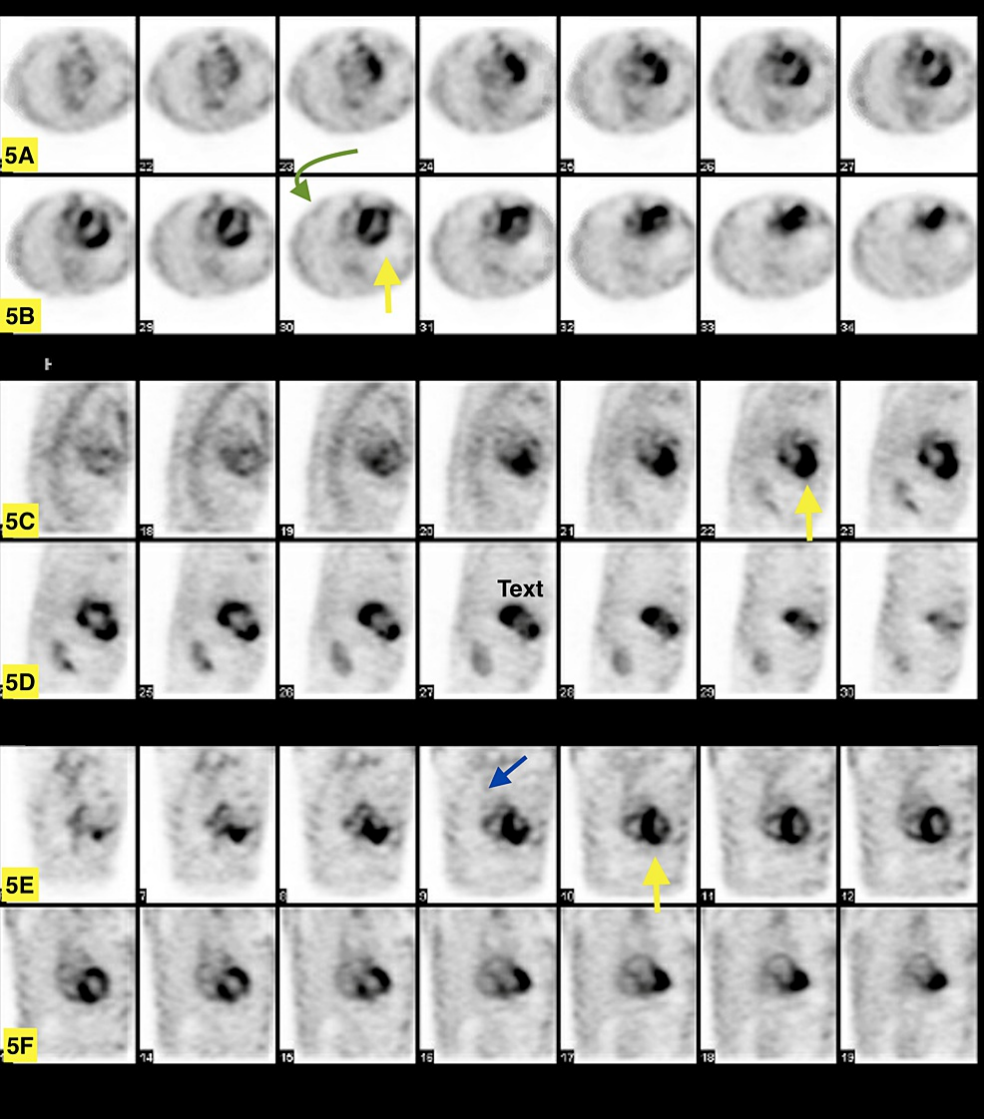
Technetium-99m pyrophosphate (Tc-99m PYP) scintigraph (5A and 5B) Head to feet transversal cut; slice thickness 6.78 mm; the heart darker than (yellow arrow) the ribs (green arrow), signalizing increased uptake. (5C and 5D) Right to left sagittal cuts; slice thickness 6.78 mm; heart signaled with a yellow arrow. (5E and 5F) Anterior to posterior coronal cuts; slice thickness 6.78 mm; heart (yellow arrow) and contralateral lung (blue arrow) signaled
